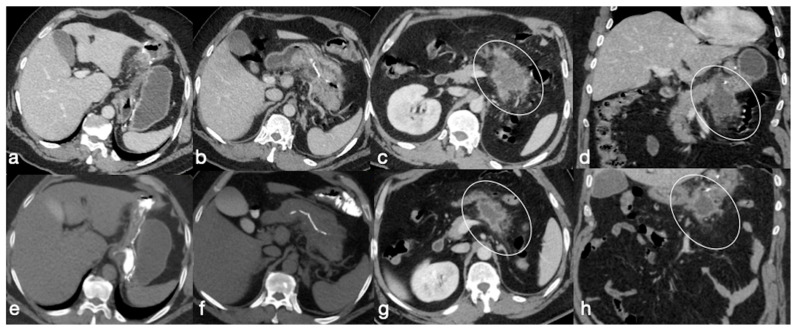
Patient 4. Leak from excluded stomach after gastric bypass. A 47-year-old male patient with previous gastric bypass was complaining of abdominal pain and fever. Note the surgical sutures of the gastric bypass (a,b, axial view) and in (c) the fluid collection (circle) that seems to arise from the excluded stomach (d, circle) and not supplied after the oral contrast administration (e,f axial views). According to the interventional radiologist and the surgeon, it was decided to conservatively treat the patients and at the CT follow-up 13 days later the fluid collection was reduced (g, axial view; h, coronal view, arrows).
